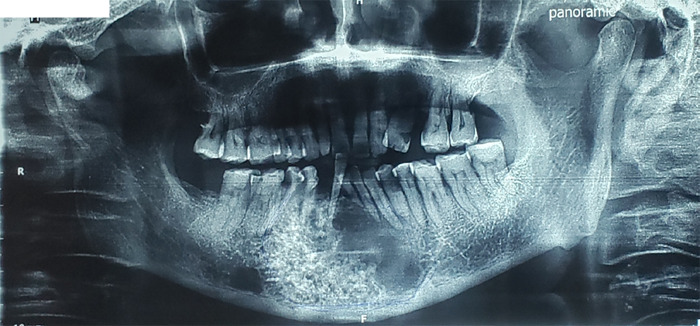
Orthopantomogram showing multilocular radiolucency with a mixed radiographic pattern of honeycomb appearance interspersed with soap bubble pattern in the right mandible.
